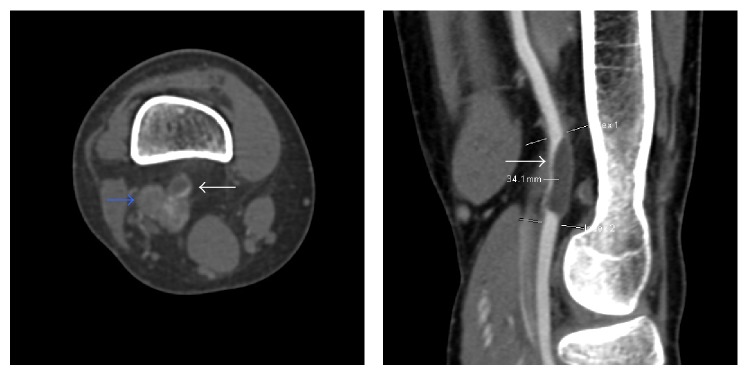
Computed tomography revealed simple cystic lesion at the popliteal artery and stenosis of the artery in this lesion. White arrow: cystic lesion of popliteal artery. Blue arrow: venous aneurysm (it was not diagnosed preoperatively).
